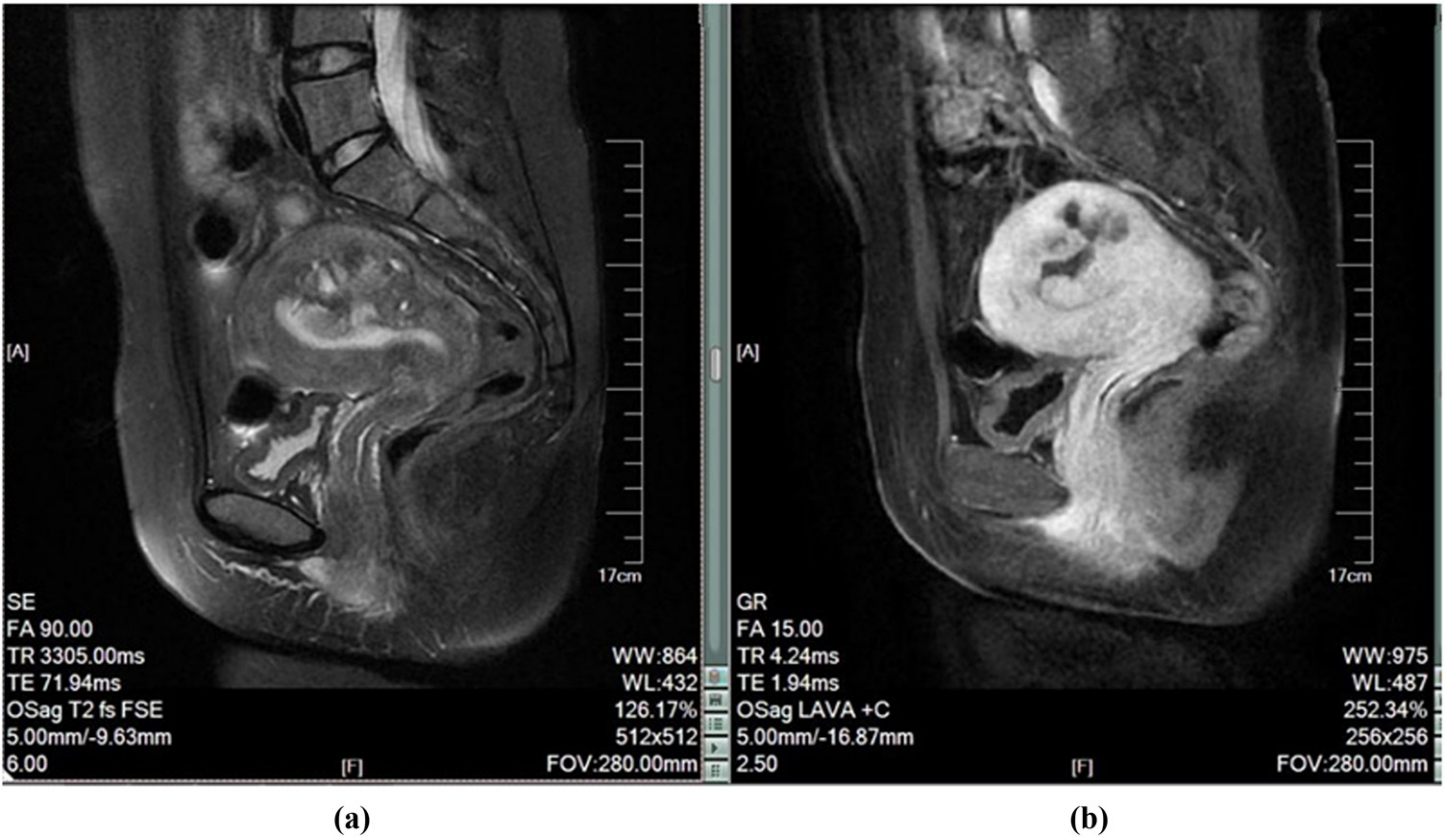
MRI image at 6 months after treatment: (a) T2 sagittal MRI image and (b) enhanced sagittal MRI image.
